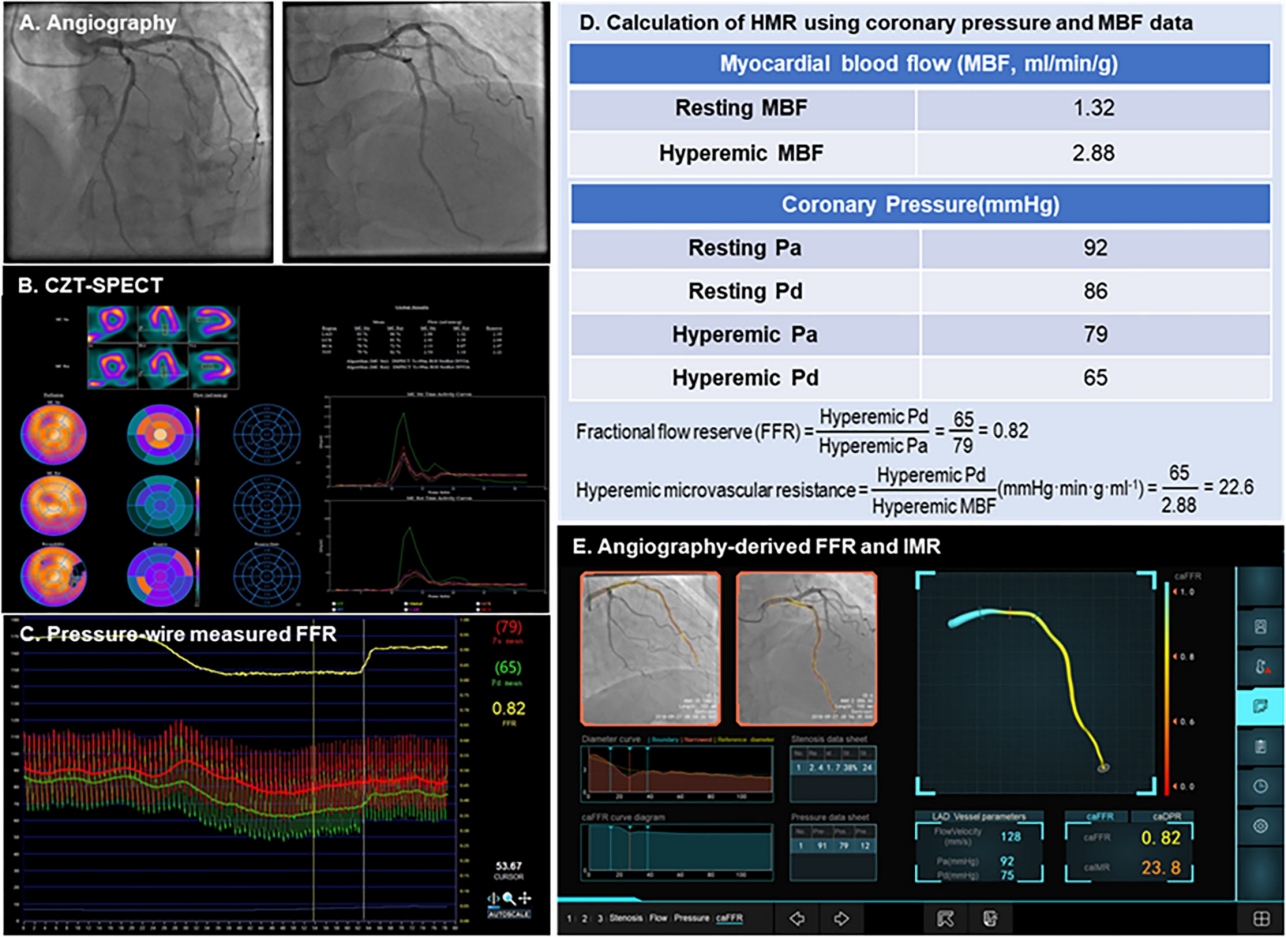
Case examples of angiography-derived physiologic indices. A representative case of CZT-SPECT MBF, pressure wire FFR, and angio-IMR measurements, as well as how HMR are calculated from MBF and coronary pressure, are shown. (A) Coronary angiography; (B) CZT-SPECT MBF; (C) Pressure wire– measured FFR; (D) Calculation of HMR using coronary pressure and MBF data; (E) Angiography-derived FFR and IMR. CZT-SPECT, cadmium–zinc–telluride single-photon emission computed tomography; FFR, fractional flow reserve; HMR, hyperemic microcirculatory resistance; IMR, index of microcirculatory resistance; MBF, myocardial blood flow.
